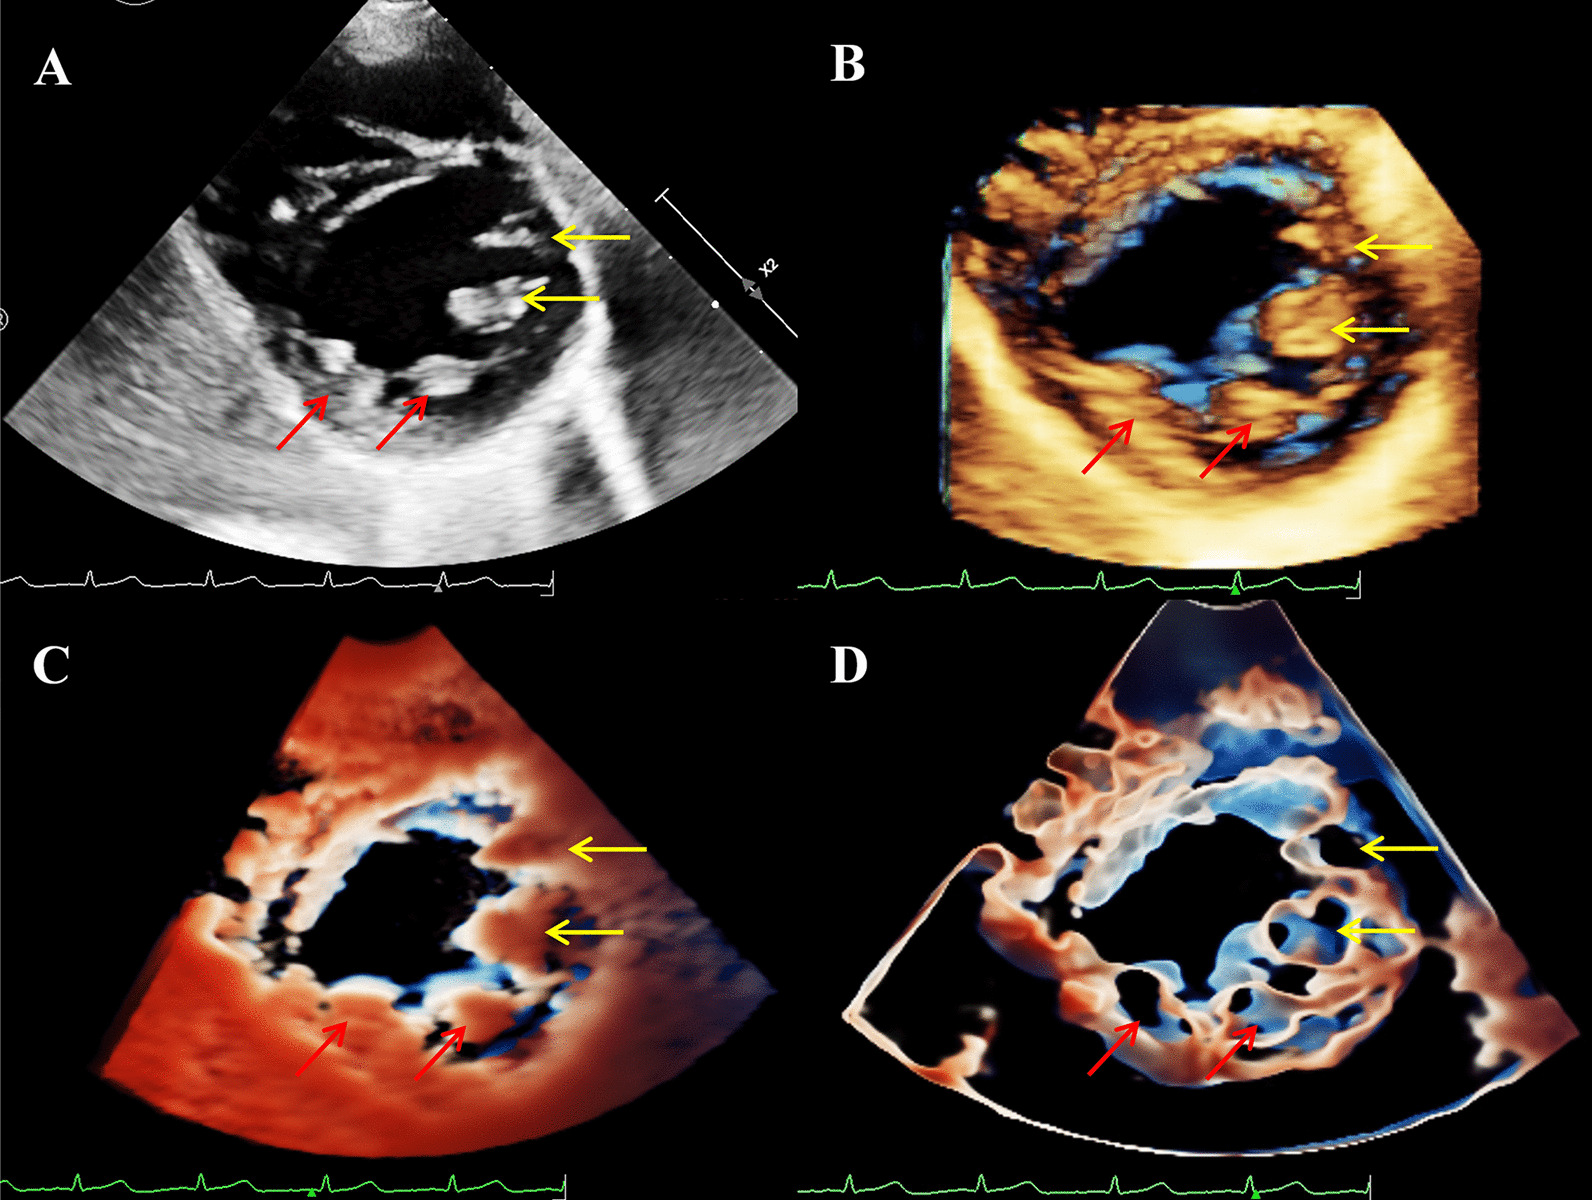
Papillary muscles. A–D 2D-TTE, traditional 3DE, TrueVue, and TrueVue Glass images showing the four papillary muscles in the left ventricular short axis section (papillary muscle level). Yellow arrows show the papillary muscles of orifice 1, red arrows show the papillary muscles of orifice 2 of the mitral valve. *2D-TTE* two-dimensional transthoracic echocardiogram, *3DE* three-dimensional echocardiography
